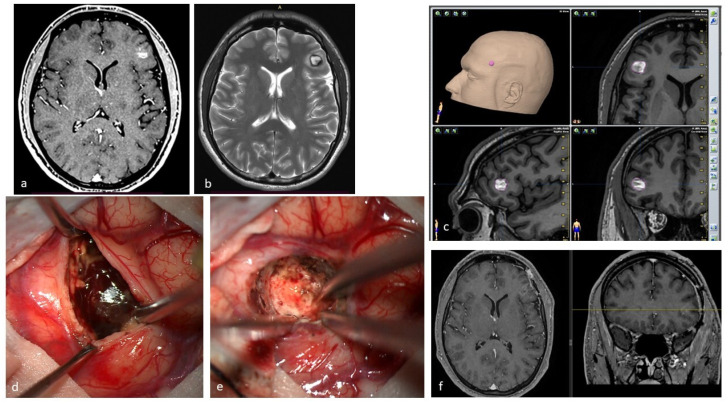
(a). Axial T1-weighted MRI with Gadolinium of a 27-year-old male affected by recurrent epileptic seizures, showing a frontal subcortical hyperdense lesion. (b). T2-weighted axial MRI showing a Type I CM according to Zabramski classification, reflecting subacute hematoma. The hypointense halo around the lesion corresponds to the hemosiderin ring. (c). Preoperative navigation planning with a T1-weighted MRI. (d). Intraoperative view showing the exposed mulberry-like CM. (e). Intraoperative view of the surgical field at the end of removal showing normal brain parenchyma without signs of the hemosiderin ring. (f). Postoperative axial and coronal T1-weighted MRI with Gadolinium showing total removal of the lesion.
